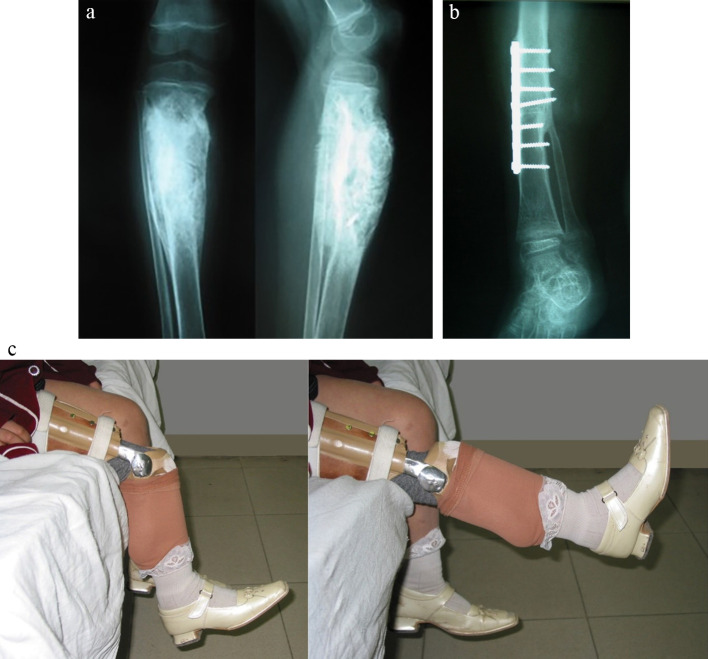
A seven-year-old girl with osteosarcoma of the proximal tibia who underwent rotationplasty type A II. Bony consolidation was seen at 3 months postoperatively. She maintained an excellent functional outcome and quality of life into adulthood with an MSTS functional score of 93.33% and a global quality of life score of 91.67%. 2-A Preoperative plain radiograph. 2-B Plain radiograph showing union of the tibia to the femoral stump. 2-C Photographs taken with the prosthesis fitted in place showing an active range of motion of the “knee” of 85°.
